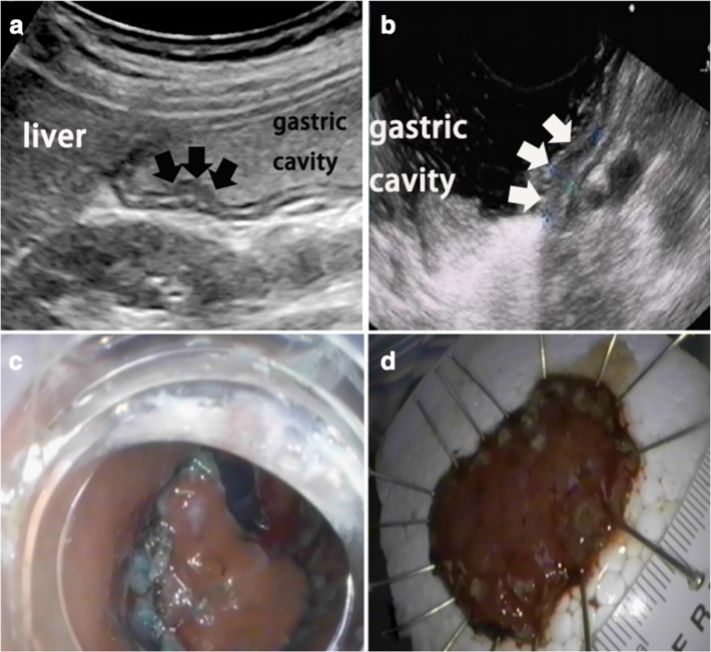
Early gastric cancer. a Examination involving transabdominal ultrasound scans after oral administration of an echoic cellulose-based gastric ultrasound contrast agent showing hypoechoic mucosal thickening of the gastric wall (*black arrows*). b Endoscopic ultrasound showing hypoechoic mucosal thickening of the gastric wall (*white arrows*). c and d Endoscopic resection of a lesion confirmed as early gastric cancer
